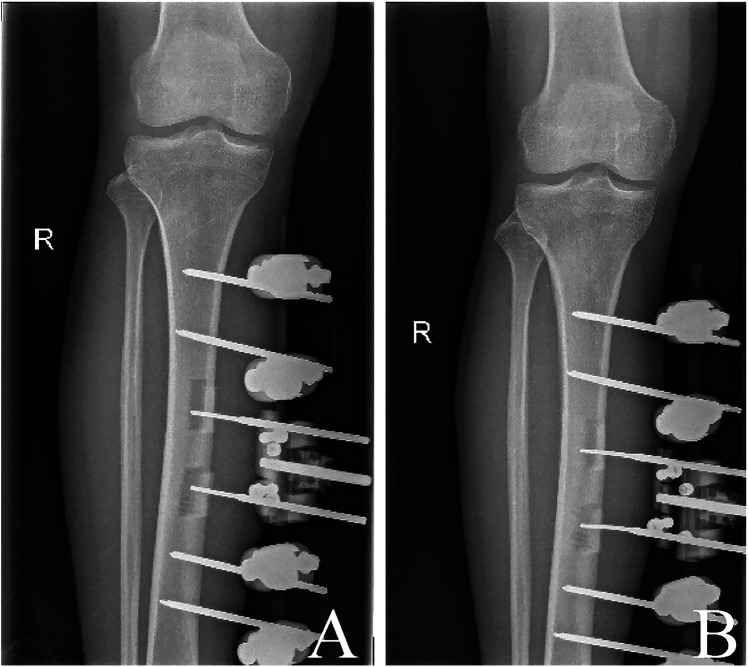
X-ray images. (A) 12d radiograph of bone distraction. (B) Radiograph on the day of removal of the external fixation frame.
